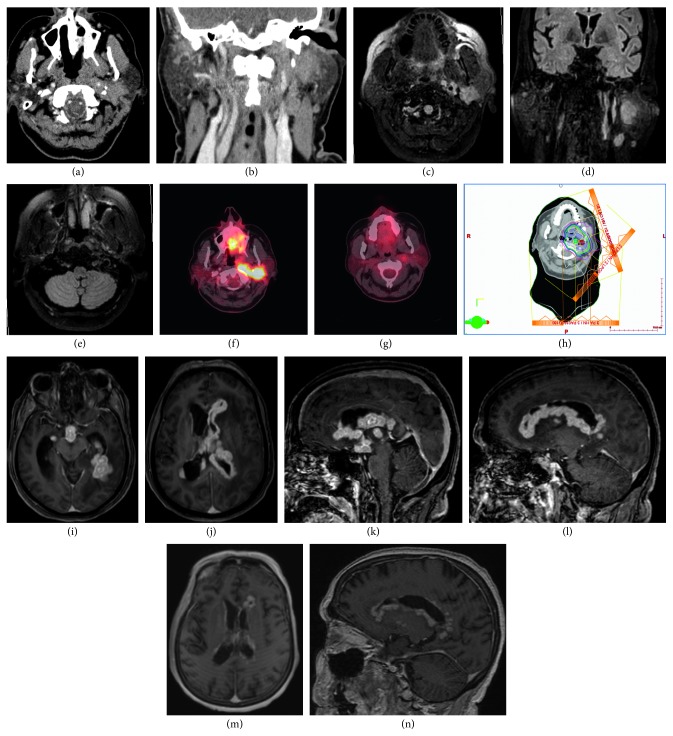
(a, b) Contrast-enhanced CT scan neck showing lobulated enhancing lesion in the deep lobe of the left parotid gland about 2.3 × 2.0 cm. Similar enhancing soft tissue thickening is seen involving adjacent left carotid space reaching up to the base of the skull. (c, d) MRI neck and brain with FLAIR images showing the same lesion in the left parotid gland and carotid space. (e) MRI brain showing involvement of the left 12th cranial nerve at the skull base. (f, g) PET CT presteroid and rituximab (July 2016) and steroid and rituximab (November 2016) showing significant resolution. (h) Proton radiotherapy. (i, j) MRI brain done in August 2017 showing extensive diffuse irregular enhancing subependymal lesions (axial views). (k, l) MRI brain done in August 2017 showing extensive diffuse irregular enhancing subependymal lesions (sagittal views). (m, n) MRI brain done in October 2017 showing significant regression of all lesions after treatment with steroid and rituximab.
